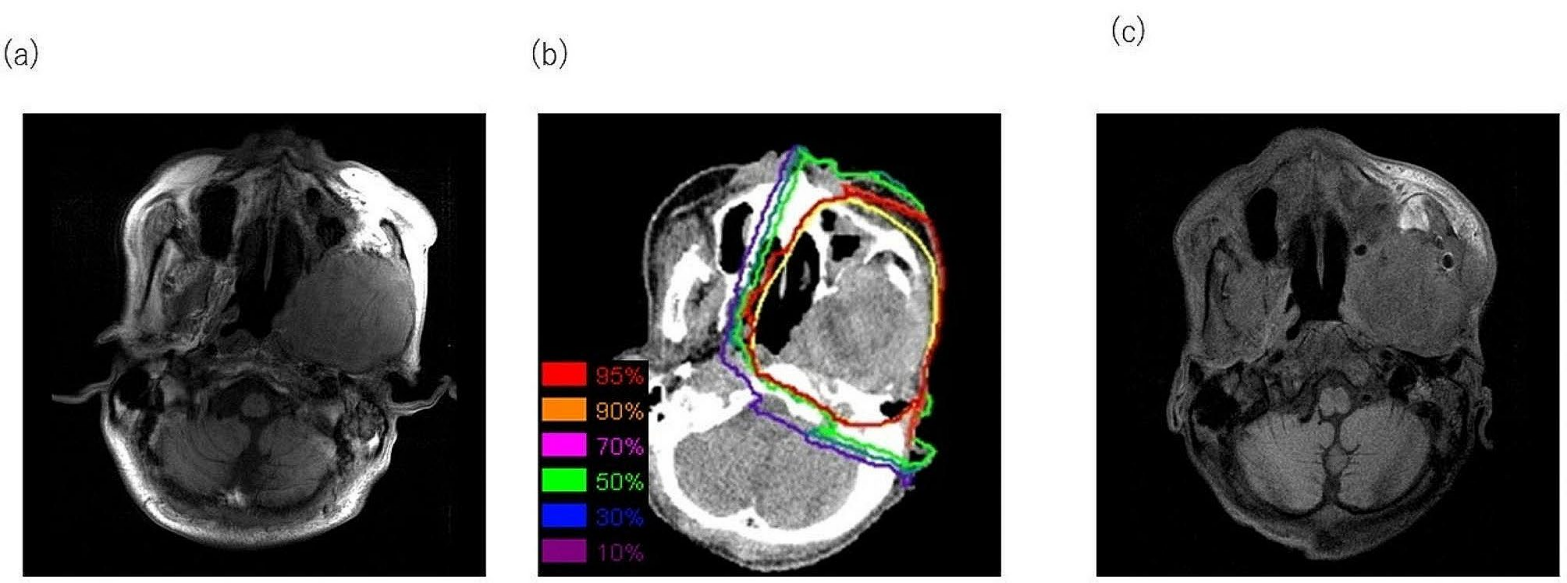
Carbon-ion radiotherapy for mandibular clear cell odontogenic carcinoma (a) Extensive clear cell odontogenic carcinoma originating from the left mandible: axial, T2 weighted magnetic resonance imaging (MRI) (b) Dose distribution of carbon-ion radiotherapy, with a prescribed dose of 57.6 Gy in 16 fractions The isodose lines correspond to 95%, 90%, 70%, 50%, 30%, and 10% dose areas. Representative computed tomography images are used to delineate planning target volume (yellow)(c) Twenty months after carbon-ion radiotherapy: no tumor growth or recurrence and stable disease in axial, contrast-enhanced T1 weighted MRI
